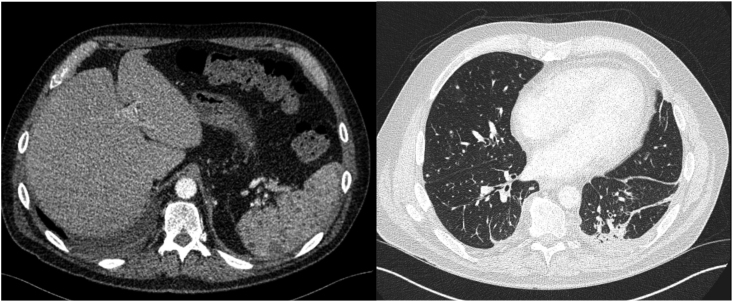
CT scan of the chest and abdomen done at Day +22 (3 weeks and 1 day) showing innumerable new bilateral pulmonary nodules and splenic lesions, suggestive of disseminated fungal infection.
